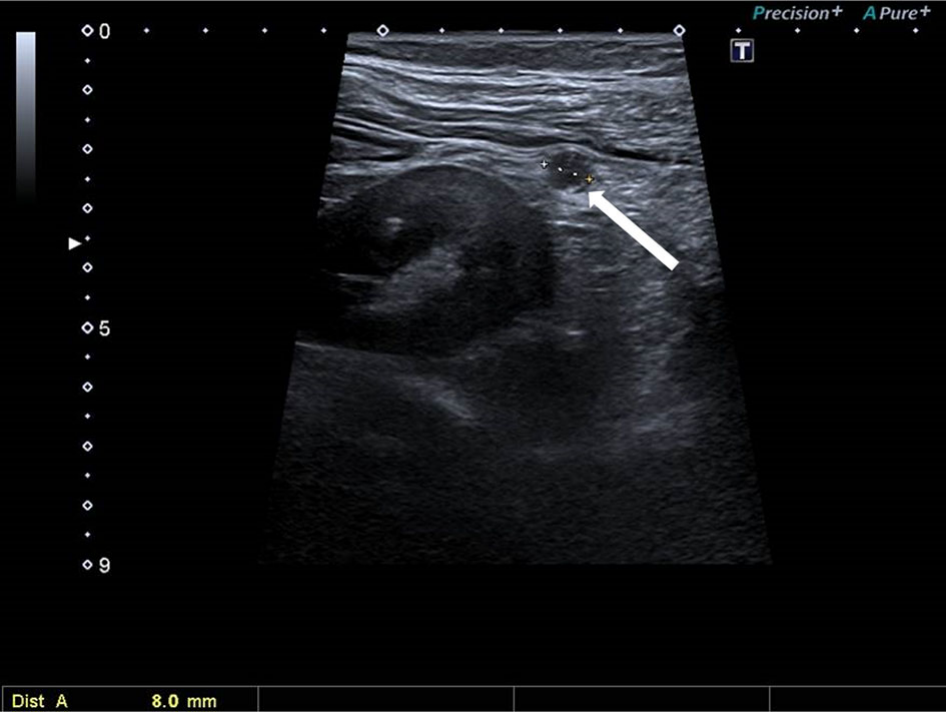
8 mm metastasis within the left perirenal fat. Ultrasonographic image.
